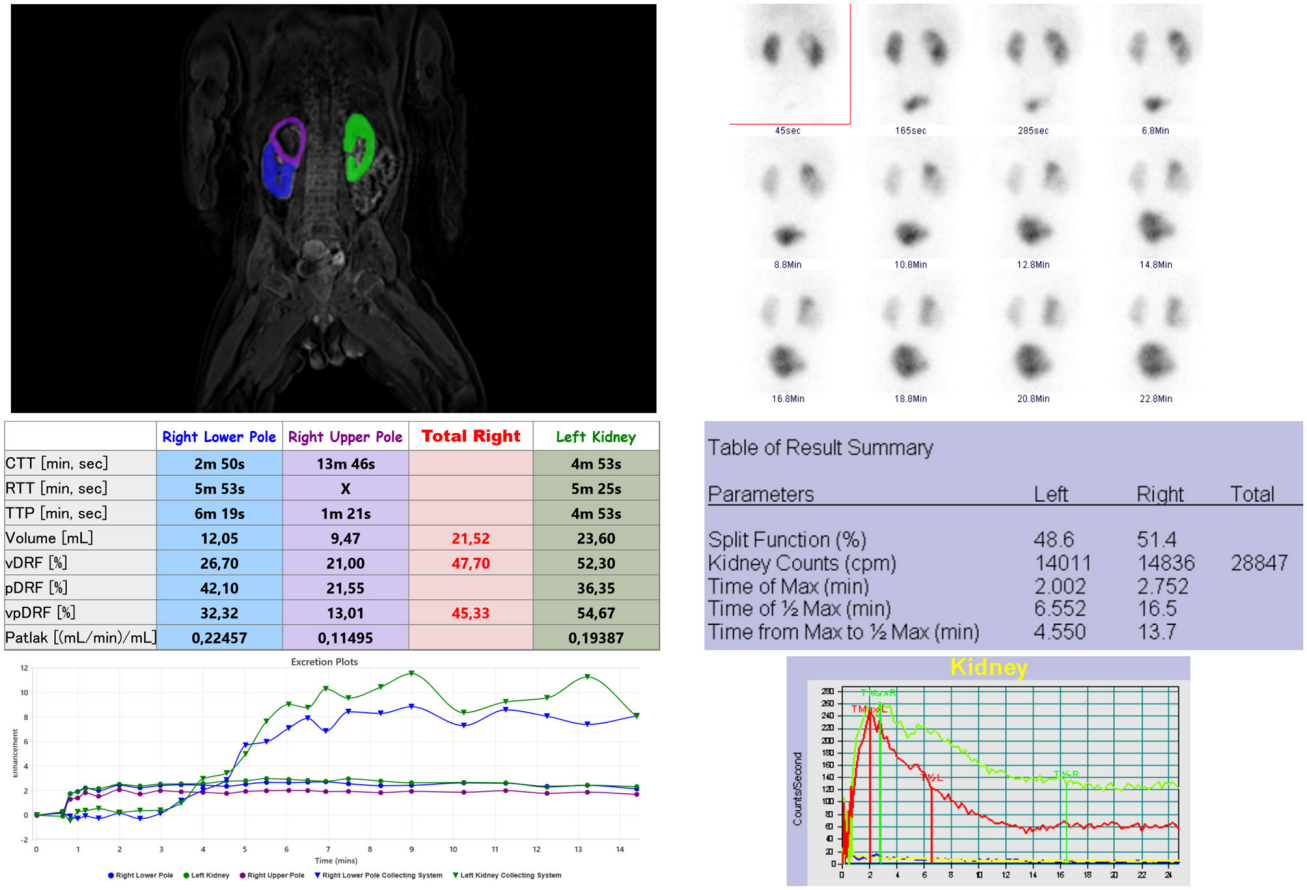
Analysis of renal functional MR urography (left) and scintigraphy (right) of a duplex right kidney with upper pole megaureter. There is graphic presentation of kidneys segmentations, absolute values of fMRU and scintigraphy parameters and at the bottom enhancement curves. In fMRU the enhancement curves and differential renal function (vpDRF) are taken separate for each moiety of right kidney. The image on the right presenting scintigraphy analysis - elongated time of T1/2Max of the right kidney due to radiotracer retention in upper pole.
